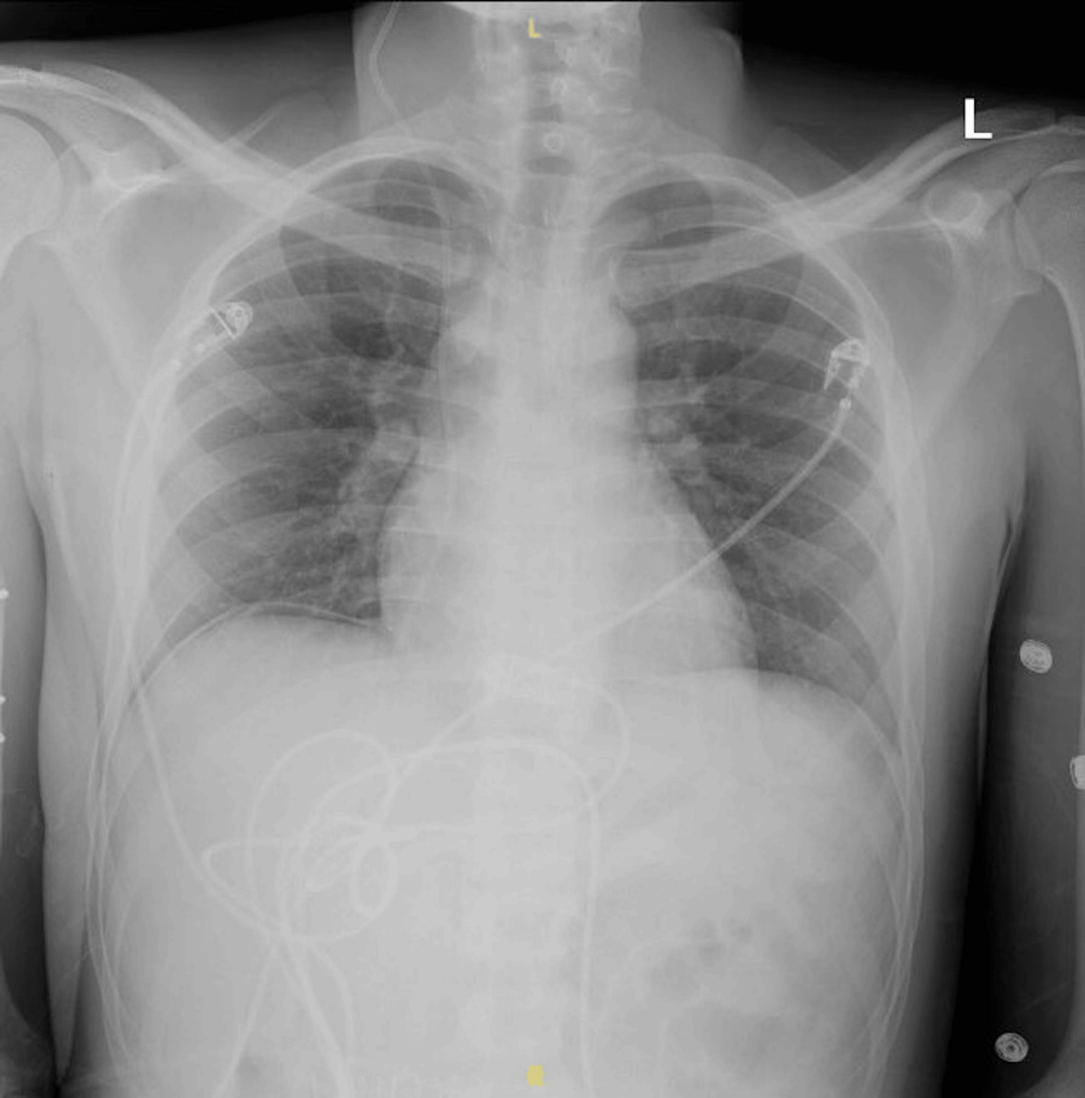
Chest radiography without any evidence of pulmonary coccidiosis
